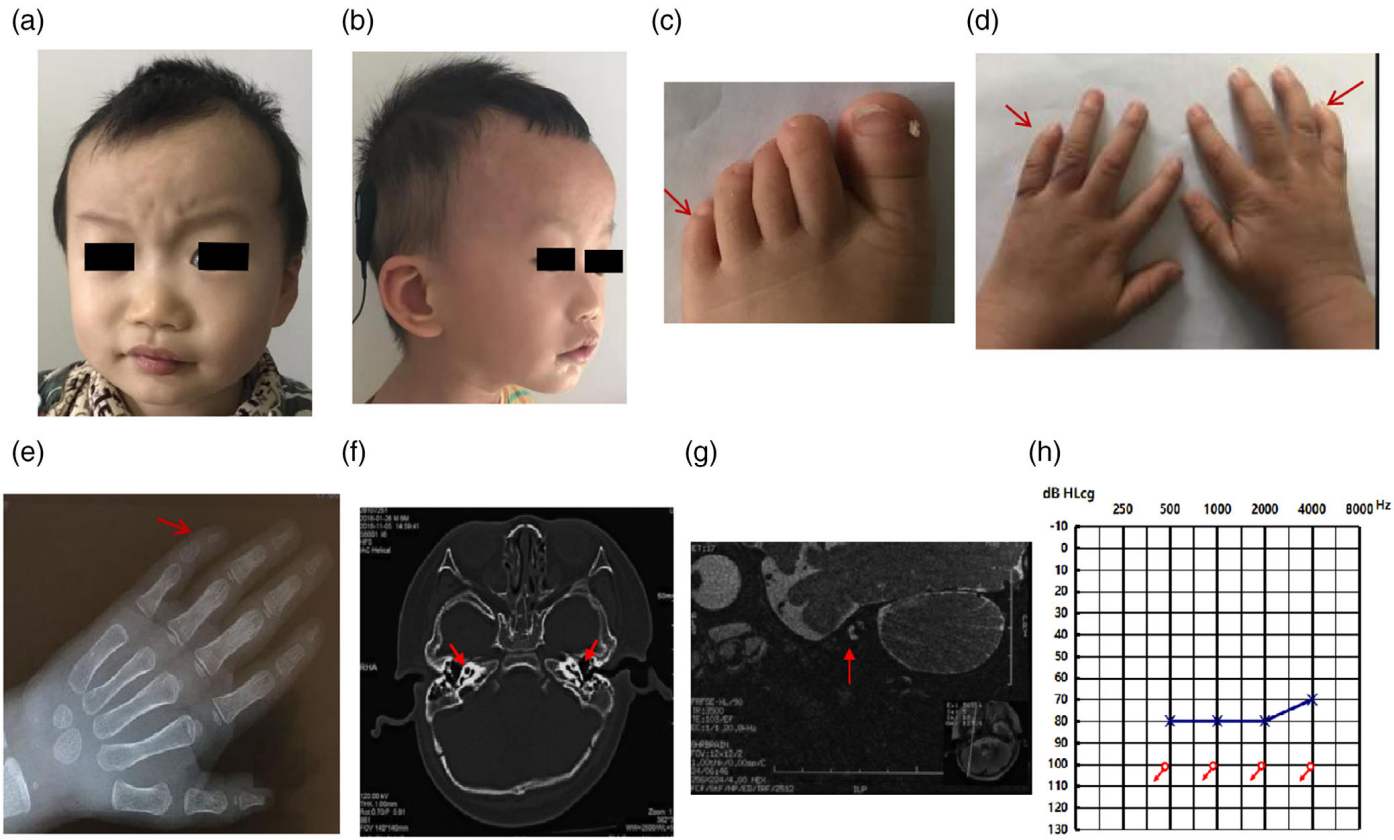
The phenotypic characteristics of proband 2. (a,b) The proband exhibited multiple unusual facial features, including high frontal hairline, sparse bilateral temporal hair, arched eyebrows, bulging forehead, wide mouth with thick lips, smooth philtrum, depressed nasal bridge, and short columella. His ears were low‐set ears with rotated backwards (c) The proband's feet exhibited hypoplastic nails, with clinodactyly of the fifth toe. (d) Bilateral clinodactyly of the fifth fingers with hypoplasia of the distal finger phalanx. (e) X‐ray image of the left hand revealed hypoplastic distal phalanges of the fifth finger. (f) A CT scan of the temporal bone revealed an incomplete partition type II cochlear malformation, with the cystic fusion of the apical turns and the middle circle. (g) Inner ear magnetic resonance hydrography failed to clearly resolve the cochlear nerve. (h) An ASSR audiogram of the proband exhibited profound sensorineural hearing loss. Blue line—left ear, red line—right ear.
